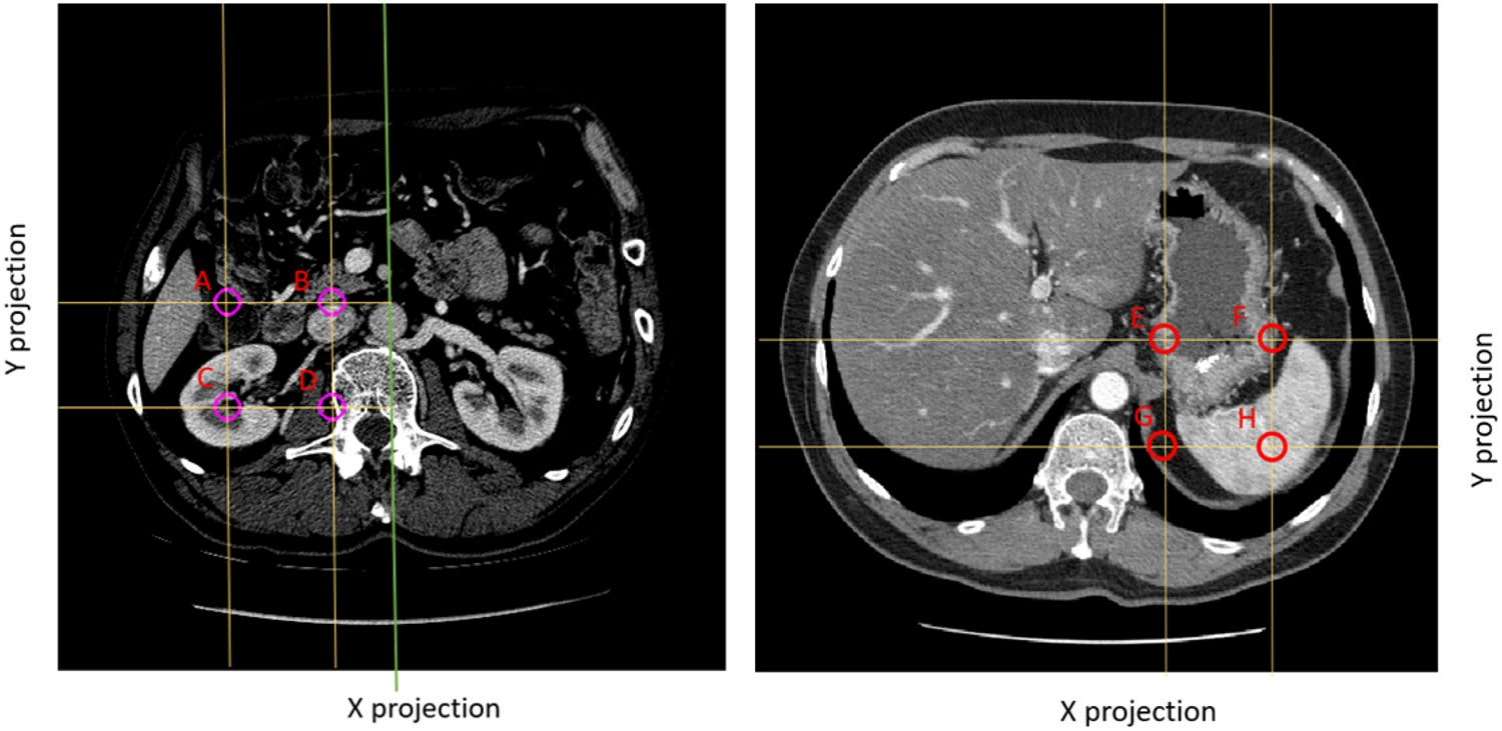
Example CT scan with superimposed points A, B, C, and D and intersecting axes in the X and Y axes. The green line crosses the center of the image.
